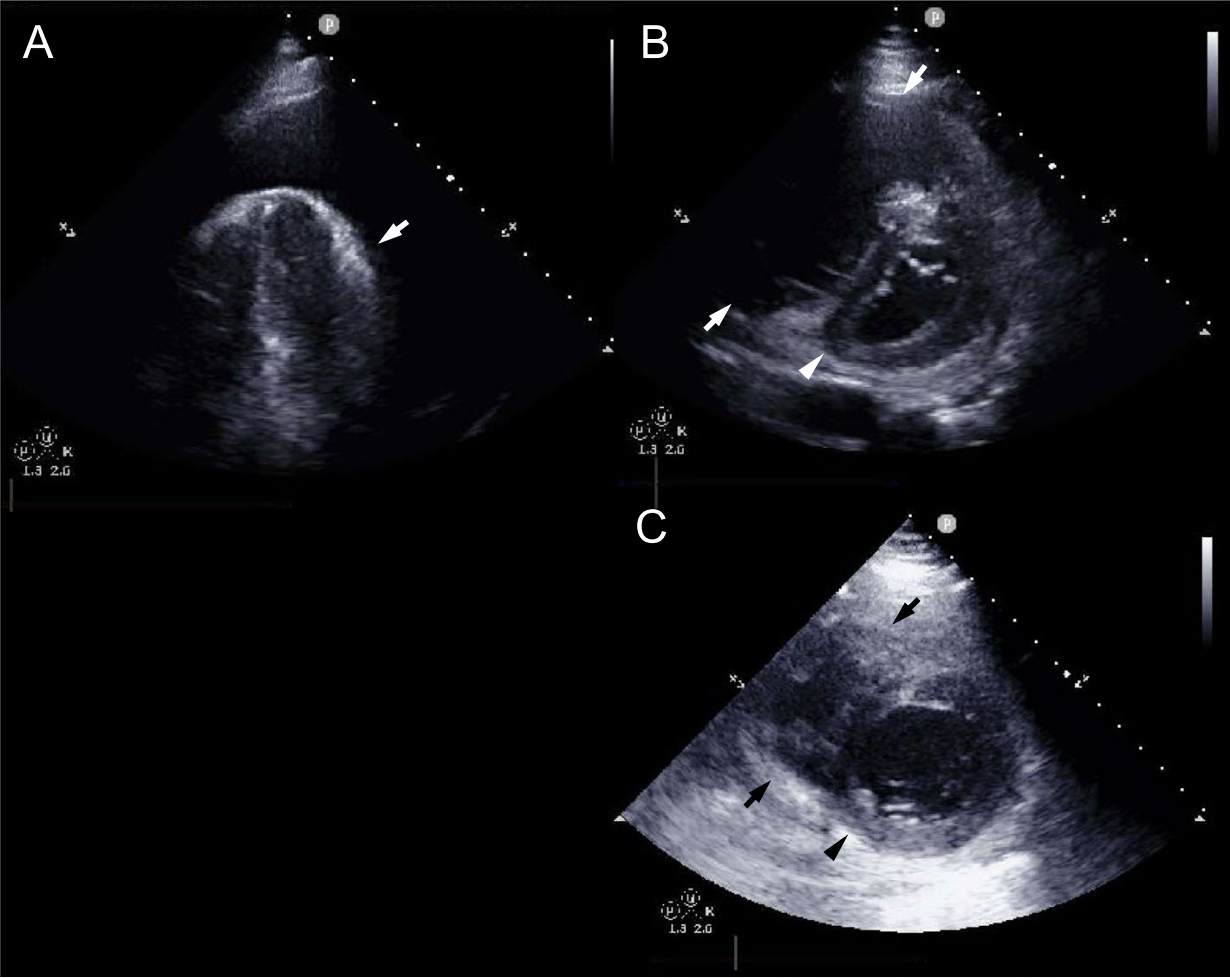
Transthoracic echocardiography (TTE) images. A Apical view obtained before pericardial drainage. The white arrow indicates the heart and the surrounding echo-free space represents pericardial effusion. B Left ventricular short-axis view at end-diastole after pericardial drainage. The pericardial effusion had resolved; however, the right ventricle became dilated (white arrows) and the left ventricle was deformed into a D-shape (white arrowhead). C Left ventricular short-axis view obtained immediately prior to decannulation, with the ECMO flow reduced to 1.5 L/min. The right ventricular size had returned to normal (black arrows). The D-shaped deformation of the left ventricle also improved and the ventricle showed a nearly circular shape (black arrowhead)
